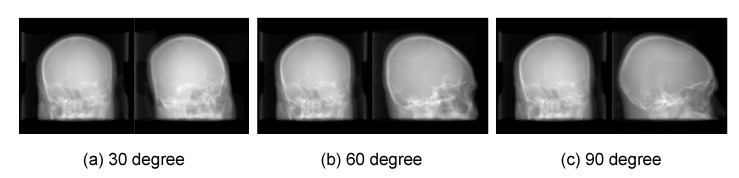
Examples of CT-DRR datasets in different projection angles.
